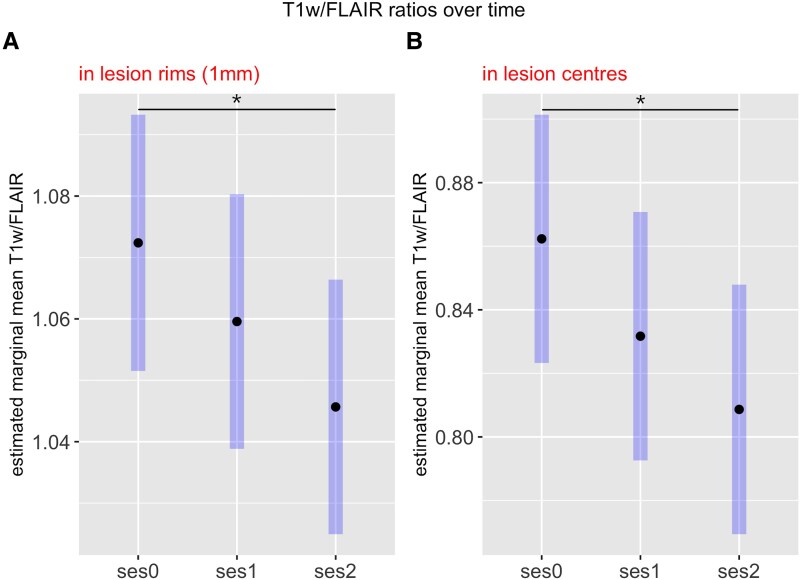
T1w/FLAIR ratios over time. Results from longitudinal analyses of T1w/FLAIR ratios in lesion rims (A) and lesion centres (B) (*N* = 39). Values were derived from linear mixed model analysis and represent estimated marginal means for baseline (ses0), follow-up after 2 years (ses1) and follow-up after 6–8 years (ses2) as bold dots and 95% confidence intervals as highlightedbars. The analysis included age (at baseline) and sex as fixed effects and subject as random effect. Significant differences (*P* < 0.05) are marked with asterisks.
